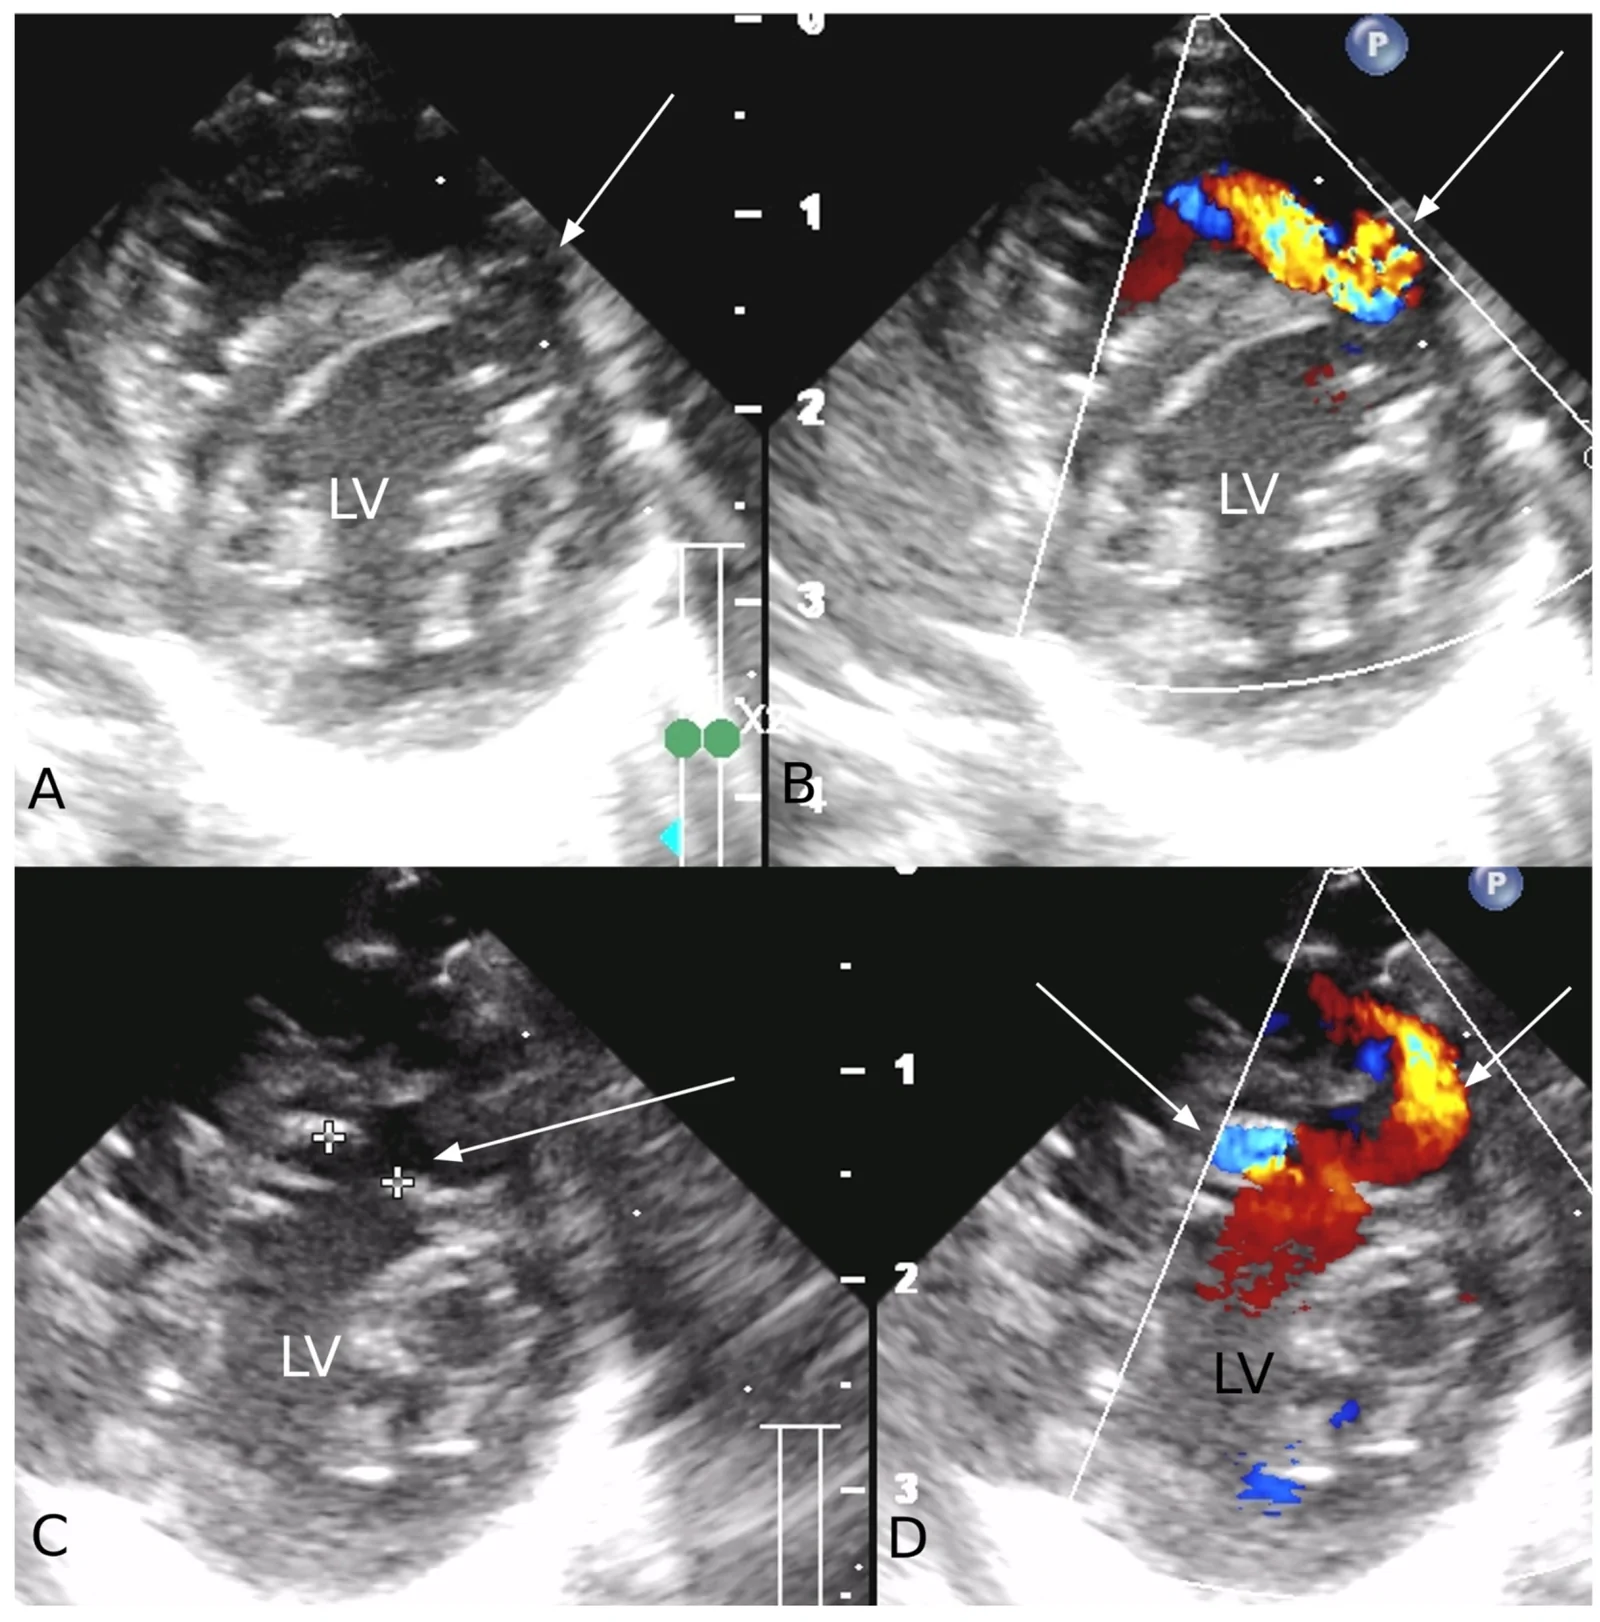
Parasternal short-axis (PSAX) echocardiography detailing multiple small muscular ventricular septal defects (VSDs) within the trabecular meshwork (white arrows). (A) B-mode evaluation showing the structural discontinuity in the interventricular septum. (B) Corresponding Color Doppler assessment demonstrating high-velocity systolic shunting jets across the defects. (C) Detailed B-mode magnification showing the exact localization and measurement of the defects using electronic calipers (white crosses, +). (D) Additional Color Doppler confirmation of the multiple distinct restrictive left-to-right shunts. LV = Left Ventricle.
